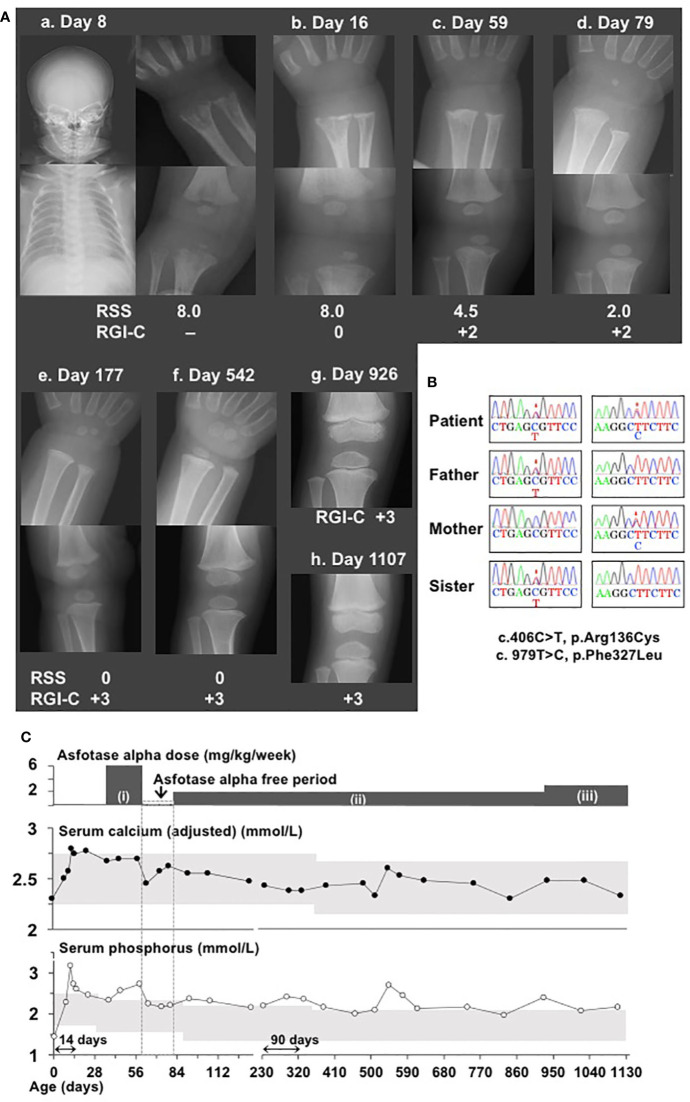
Clinical and molecular findings in a patient with hypophosphatasia. (A) X-ray examinations of the wrist and knee showed typical features of hypophosphatasia, whereas the head and chest showed no abnormal features (a and b). X-ray examinations of the wrist and knee after initiation of asfotase alfa (AA) therapy with the standard dose (2 mg/kg, three times per week = 6 mg/kg/week) showed substantial improvement of rickets (c. 59 days of age). Temporary interruption of AA therapy did not worsen skeletal features, as indicated by reduction of Rickets Severity Scale score from 4.5 to 2.0 and maintenance of Radiographic Global Impression of Change rating +2 (d). Lower doses of AA (2 mg/kg/week) maintained normalized skeletal features (e and f). X-ray examination of knee revealed slight distal metaphyseal demineralization (g); increased dose from 2 mg/kg/week to 2.4 mg/kg/week led to recovery of mineralization (h). “Day” represents days of age. (B) Electrochromatography showed a compound heterozygous missense mutation of *TNSALP* (c.406C>T, p.Arg136Cys and c.979T>C, p.Phe327Leu) in this patient, as well as a heterozygous missense mutation at c.406C>T in her father and sister, and a heterozygous missense mutation c.979T>C in her mother. Mutations are indicated by asterisks. (C) Clinical course of disease in this patient. Upper graph shows changes in serum calcium levels after Payne’s correction (13). Lower graph shows changes in serum ionic phosphate levels. Gray areas indicate age-matched reference ranges. AA therapy at the dose of (i): 6 mg/kg/week, (ii): 2 mg/kg/week, and (iii): 2.4 mg/kg/week. Monitoring frequencies differed among clinical phases, such that monitoring was performed at 4–7-day intervals during pretreatment, initiation of therapy, and interruption of therapy; at 2–4-week intervals during resumption of therapy at a reduced dose; and at 2–3-month intervals during follow-up. Thus, the horizontal line axis from birth to 134 days of age was set to a period of 14 days; from 214 to 1,108 days, it was set to be a period of 90 days. “Day” represents age in days.
